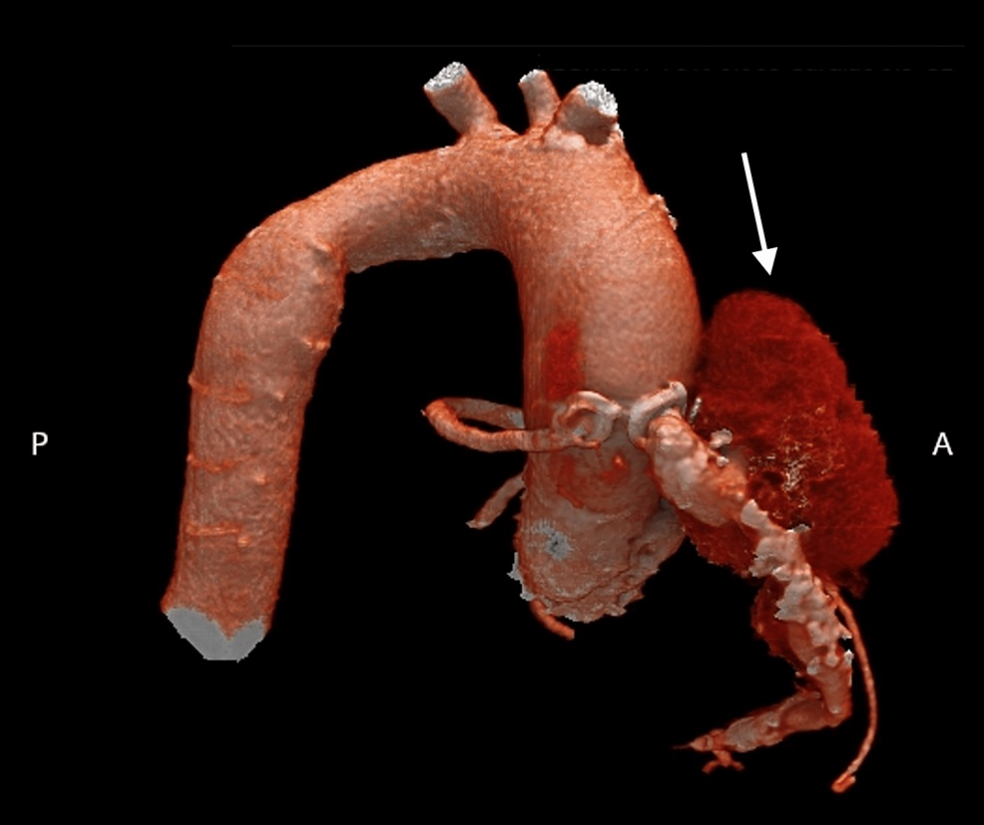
Pre-Stent deployment CT 3D volume-rendered imaging Computed tomography three-dimensional volume render scan showing a pseudoaneurysm in the saphenous vein graft (SVG) to the right coronary artery (RCA), measuring 5.7 cm in size.
